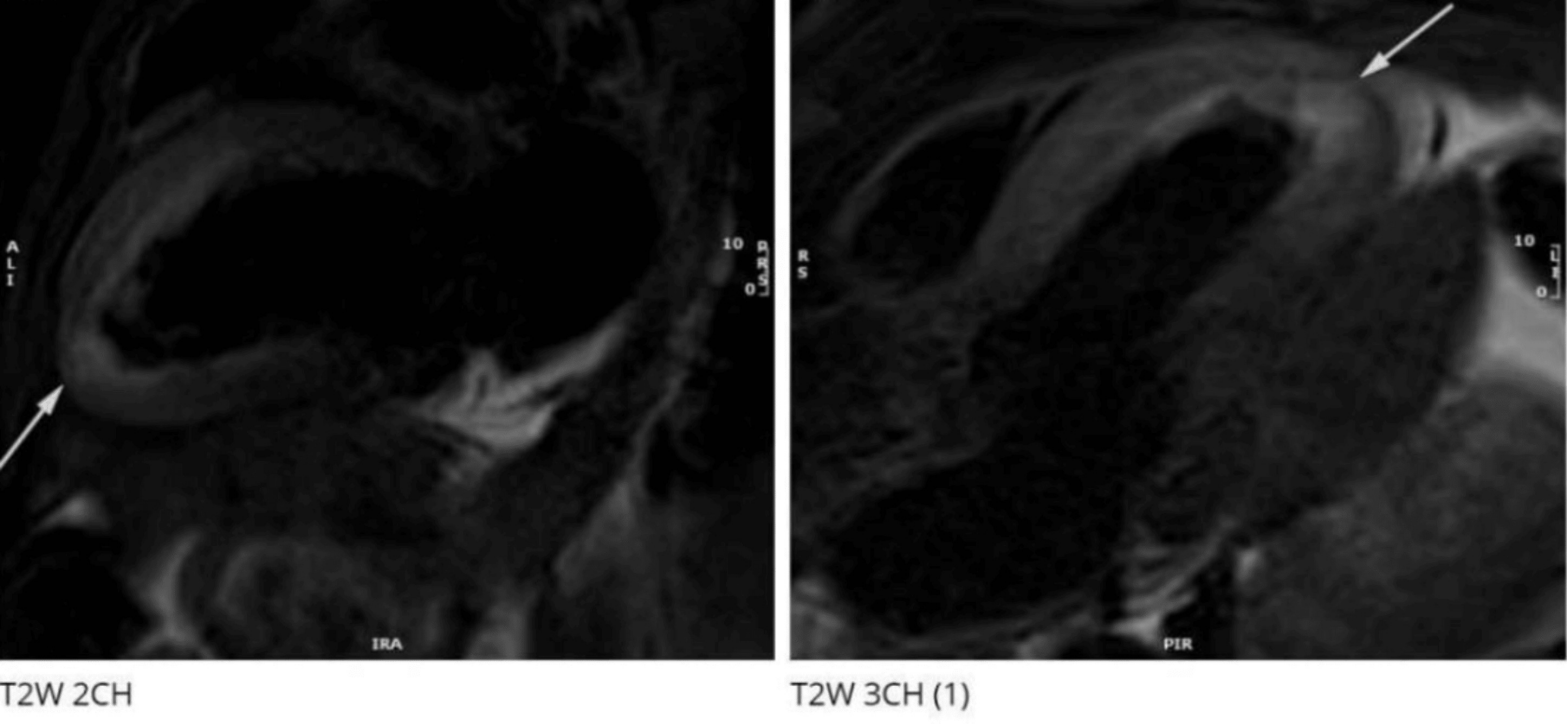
Cardiac MRI two-chamber (2CH) and three-chamber (3CH) views showing myocardial infarction in the left anterior descending artery territory. T2-weighted imaging demonstrated increased myocardial signal intensity within the mid to apical anterior wall (arrow) in the 2CH view and similar increased signal intensity in the mid to apical anteroseptum and apical cap (arrow) in the 3CH view.
